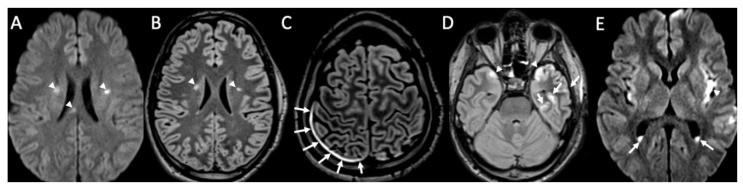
Axial DWI (A,E), FLAIR (B), enhanced FLAIR (C,D), show multiple recent ischemic areas (arrowheads in A,B,D) in a patient with Neisserria Meningitidis infection. Note also pachymeningeal (arrows in C) and leptomeningeal enhancement (arrows D) and purulent material in the subarachnoid spaces in the left insular region (arrowhead in E) and in the lateral ventricle bilaterally (arrows in E).
